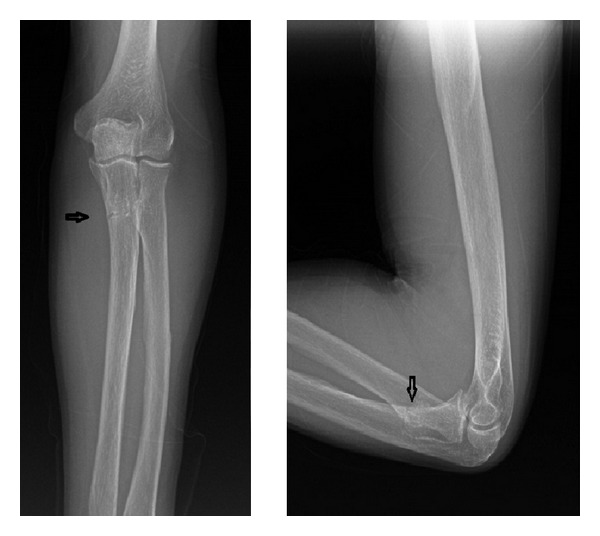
Anteroposterior and lateral X-rays showing stress fracture in proximal metaphysis of left ulna.
